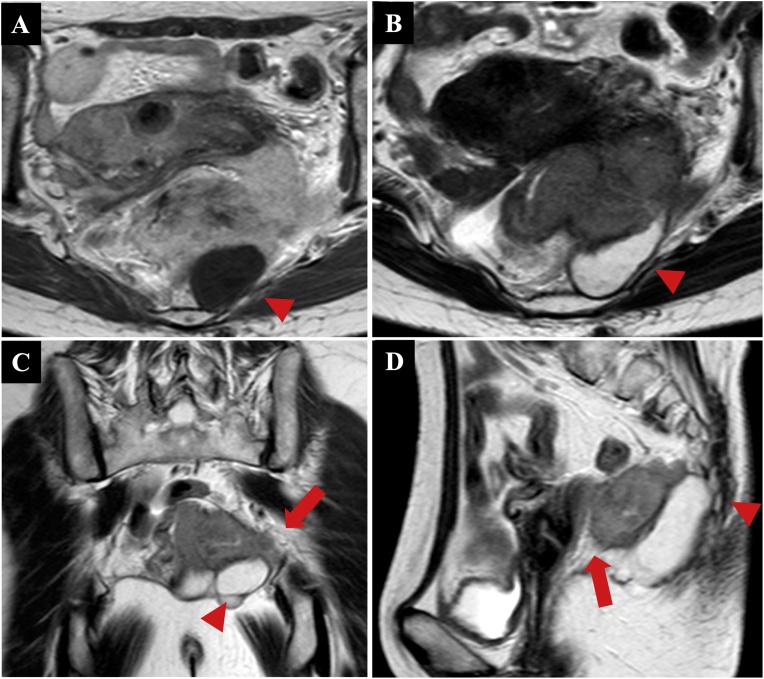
T1-weighted magnetic resonance image shows a unilocular cyst and marginated low-intensity mass (arrowhead) (A). T2-weighted magnetic resonance image shows a high-intensity mass (arrowhead) (B). A solid mass with a contrast effect is found on the left side and head side of the cyst in the T2-weighted magnetic resonance image. The solid part touches the rectum, vagina, and left sciatic spine, with possible invasion (arrow). The cyst does not involve the levator ani muscle in the coccyx and sacrum (arrowhead) (C: Coronal section, D: Sagittal section).
